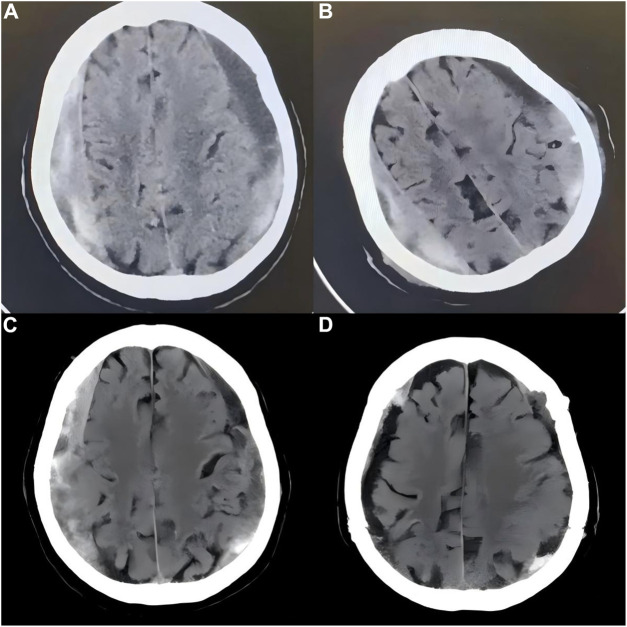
(A) CT scan performed before the initial burr-hole drainage surgery 2 years prior, showing hyperdense areas. (B) CT scan performed 1 week after the initial burr-hole drainage procedure, showing persistent bilateral subdural collections. (C) Preoperative cranial CT scan before craniotomy, showing bilateral frontotemporoparietal chronic subdural hematomas of inhomogeneous density, including hyperdense areas and a significant mass effect. (D) Follow-up CT scan on postoperative day 40, showing good re-expansion of the brain parenchyma in the operative area.
